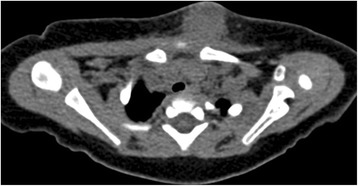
The chest CT shows a 2 x 3 cm ill-defined soft tissue density lesion with abscess formation, adjacent infiltration into subcutaneous fat and the pectoralis major muscle. Also there is no lesion in the pulmonary parenchyma.
